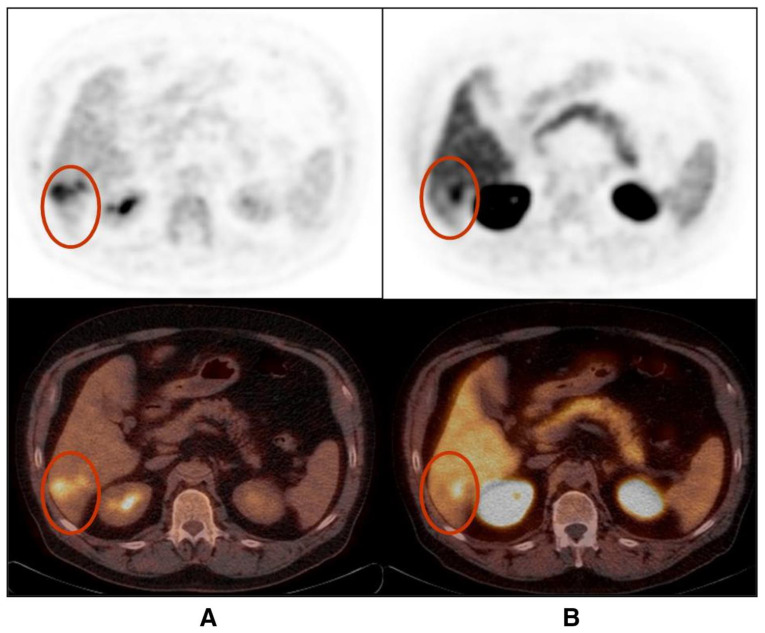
Poorly differentiated hepatocellular carcinoma shows partially congruent uptake of FDG (A) and FCH (B) (lesions shown in red circles). The FDG-positive areas appear photopeanic on FCH PET/CT (images reproduced with permission from [53]).
